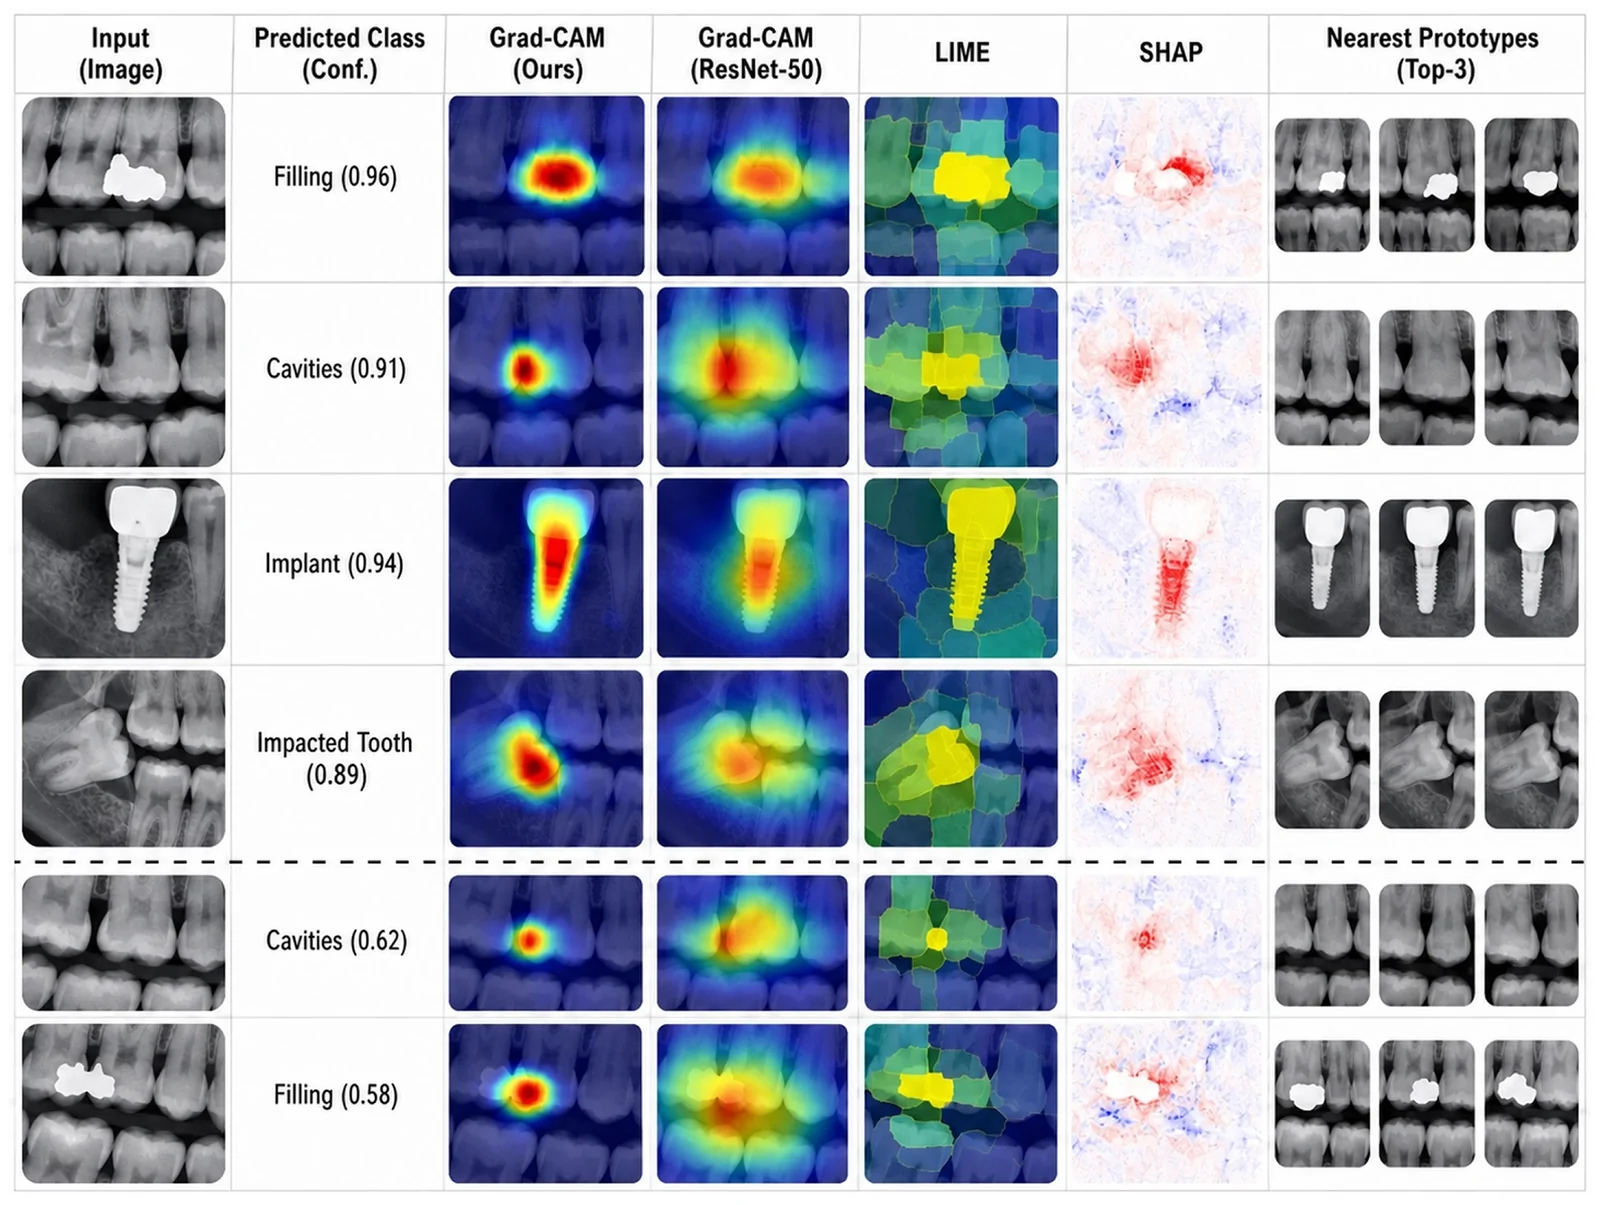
Qualitative comparison of visual explanation methods, attribution maps, and top-3 retrieved prototypes for representative dental radiographs.
